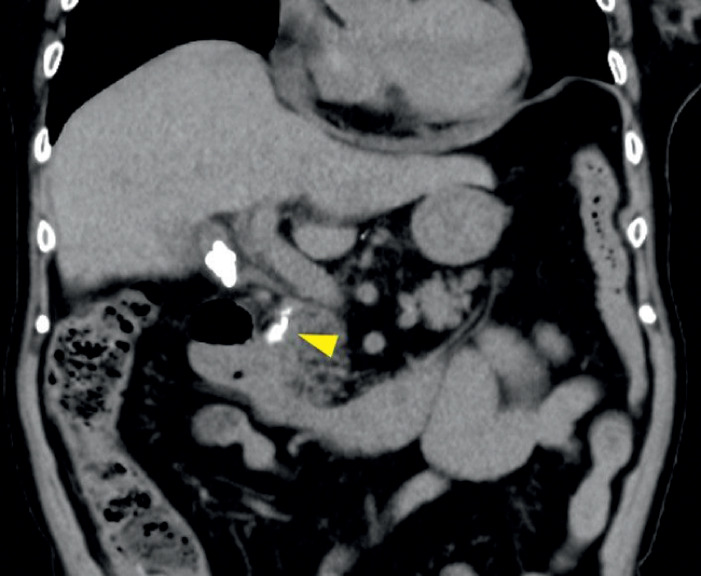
Computed tomography (CT) images. CT revealed a common bile duct stone.
